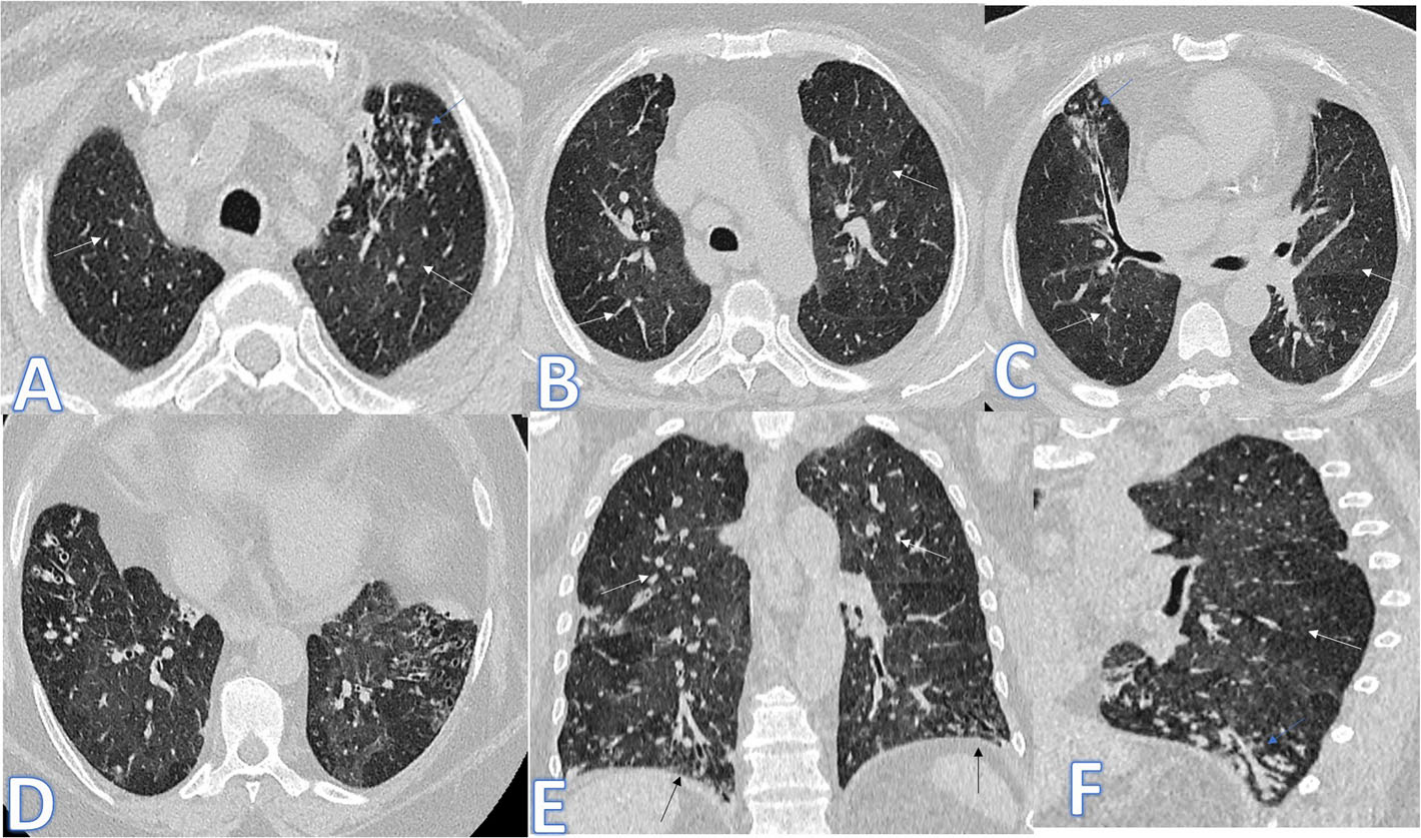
A 61-year-old female patient with bronchial asthma presented with cough and wheezy chest. On CT, there were bilateral mosaic patches of GGO due to air trapping (white arrows) with left upper lobe tree-in bud and subsegmental atelectasis (blue arrows), peribronchial thickening, and bilateral mild basal bronchoectatic changes (black arrows)
